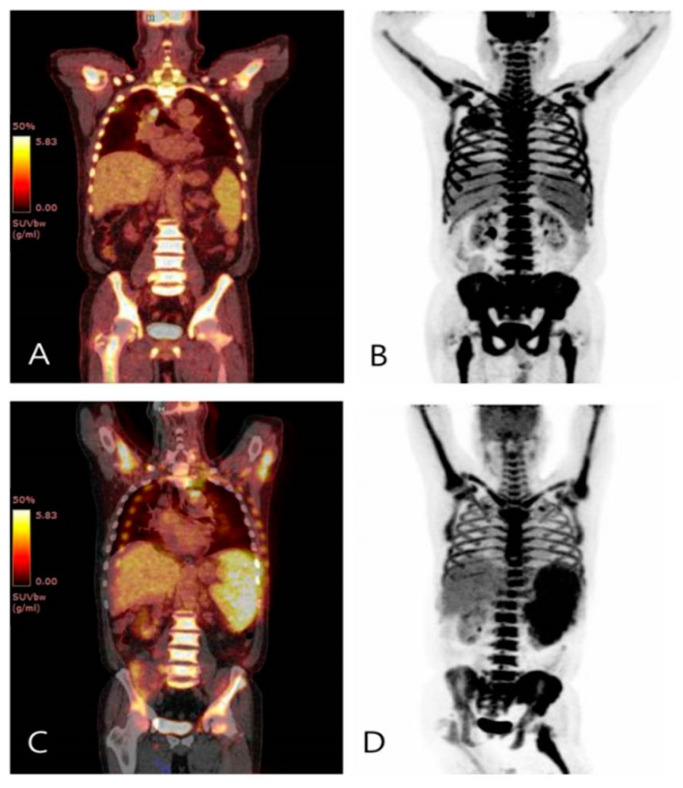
Coronal (A) and maximum-intensity projection (MIP) (B) F-18 FDG PET/CT images showed marked increase in FDG uptake in the bone marrow spaces (SUVmax 10.36). Additionally, abnormal FDG uptake in peribronchial consolidation of right lung suggests active inflammatory lesion. No other significant increase in FDG uptake suggesting malignancy was detected. A further increase in metabolism was demonstrated on coronal (C) and MIP (D) PET/CT images 3 months after the initiation of treatment, with SUVmax 12.17 for bone marrow and 7.17 for spleen.
